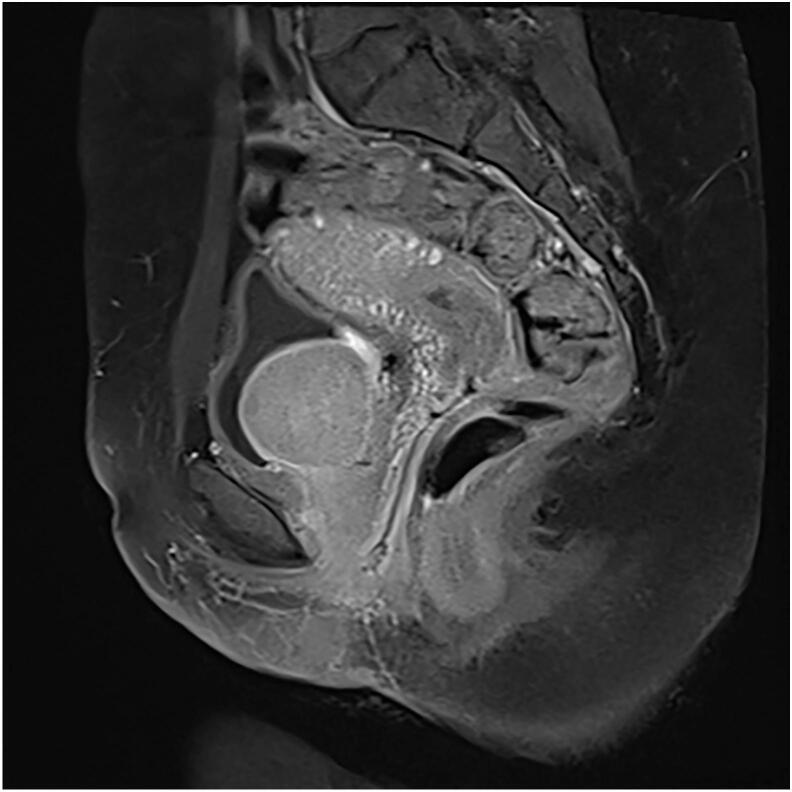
T2 weighted, MRI sagittal view, revealed a well-circumscribed intramural tumor on the left side measuring 4.3 cm × 4.2 cm.
